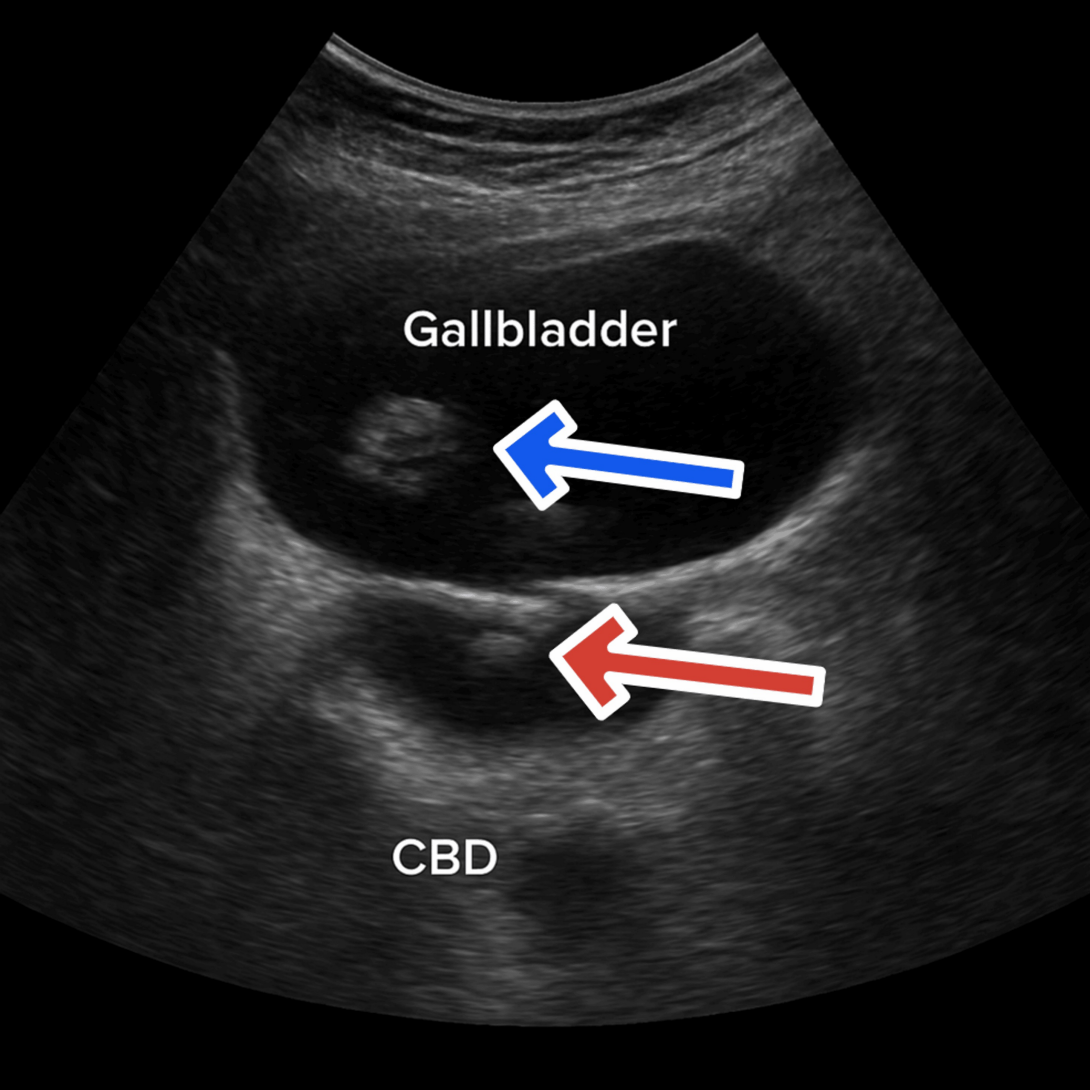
Ultrasound of the abdomen showing the distended gall bladder and common bile duct with multiple calculi (Oct 21, 2024) Abdominal ultrasound demonstrating a distended gallbladder containing multiple calculi (aggregate size up to 15 mm) with mild wall thickening as shown by the blue arrow. The common bile duct (CBD) is dilated to 12 mm, showing an echogenic focus consistent with an obstructing 6 mm CBD stone (red arrow). No pericholecystic fluid or intrahepatic biliary dilation is observed. These findings support the diagnosis of acute biliary pancreatitis secondary to choledocholithiasis.
